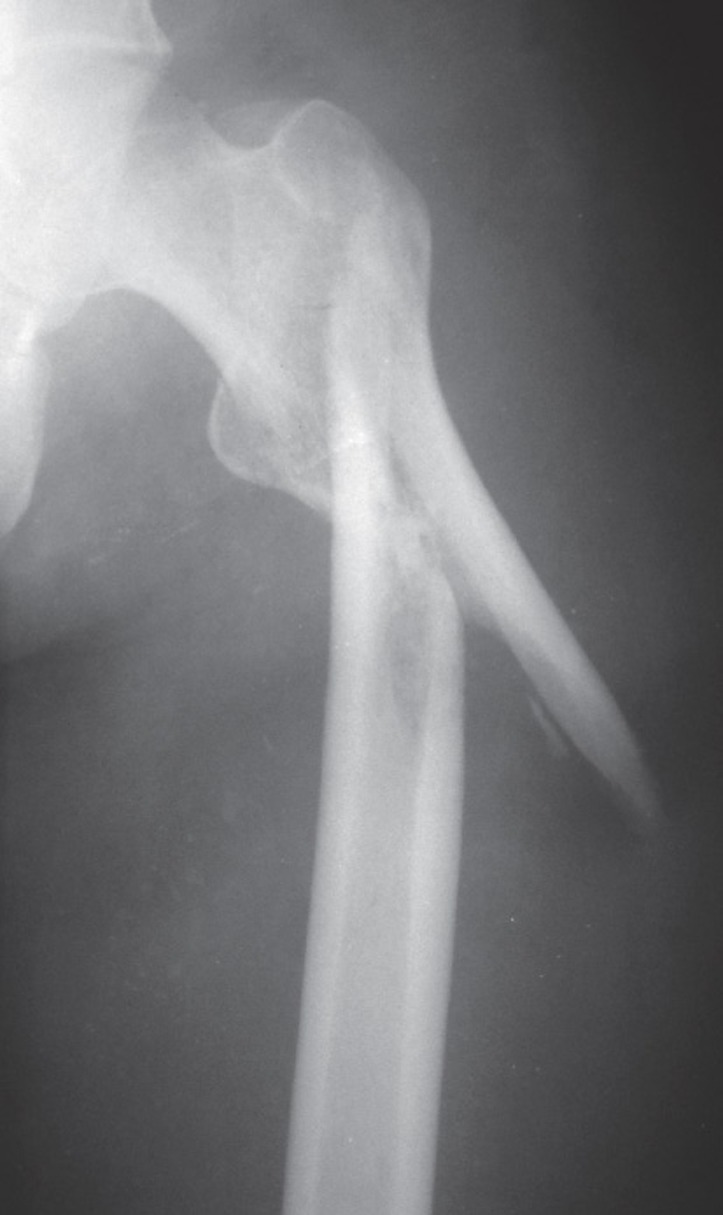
After 3 months on skeletal traction. Yet again, a lateral radiograph was not possible because of lack of resources. There was no obvious callus formation. There was some rounding of the sharp fragment ends and the displacement had increased.
